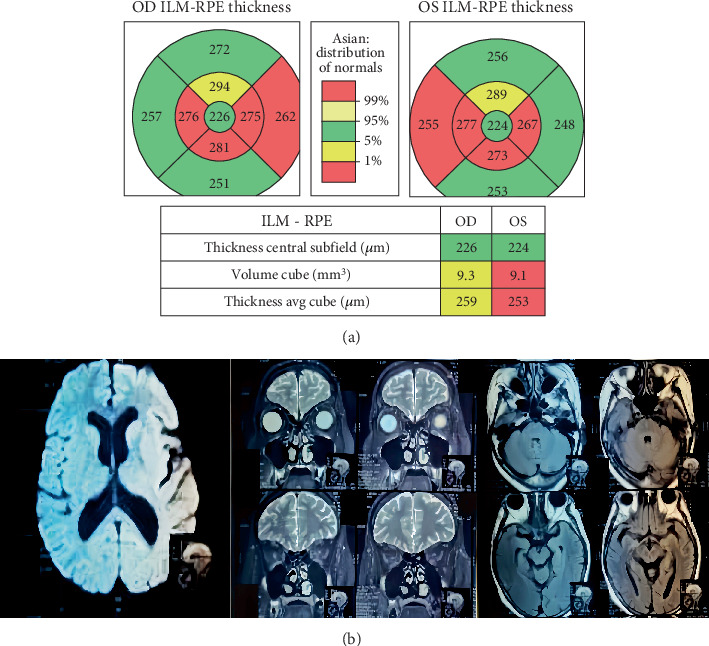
(a) Abnormal macular cube. (b) MRI head focus orbital axial slice.
